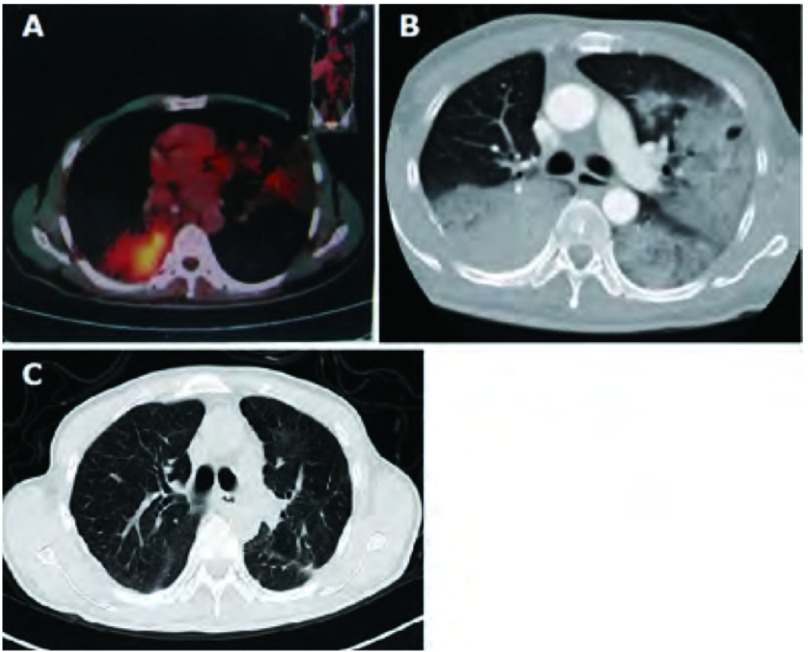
患者临床影像学特征。A：术前PET-CT示双肺多发斑片状及团块样浸润阴影，纵隔淋巴结不肿大，其余部位未见转移；B：术前胸部CT示双肺野多发大片状密度增高影，边缘模糊，左肺见多个结节影；C：术后6个月随访复查胸部CT显示双肺复张良好，未见明显肿瘤复发。 Clinical radiologic features of the patient. A: Preoperative PET-CT showing bilateral multiple nodules and mass without positive lymph nodes or extrapulmonary metastasis; B: Preoperative chest CT scan showing diffuse bilateral ground-glass opacities and nodules; C: CT scan of the chest obtained 6 months postoperative showing satisfactory reexpansion of double lungs with no evidence for recurrence.
